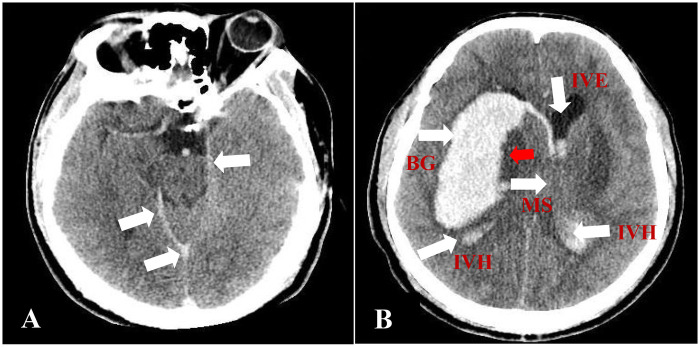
(A) Initial head CT (brain window settings: width 85 HU, level 35 HU) showing subarachnoid hemorrhage with high-density shadows along the tentorium cerebelli, longitudinal fissure, cerebral falx, and sulci (white arrows). (B) Follow-up CT 15 h later demonstrating massive right basal ganglia hemorrhage (9.0 × 3.3 cm) centered in the right putamen and caudate nucleus (labeled), with surrounding hypodensity consistent with necrotic change (arrowheads), intraventricular extension, and significant midline shift. The second image clearly demonstrates the classic methanol-associated hemorrhagic necrosis of the basal ganglia. Anatomical structures labeled: BG, basal ganglia (putamen and caudate nucleus); IVH, intraventricular hemorrhage; IVE, intraventricular extension; MS, midline shift.
